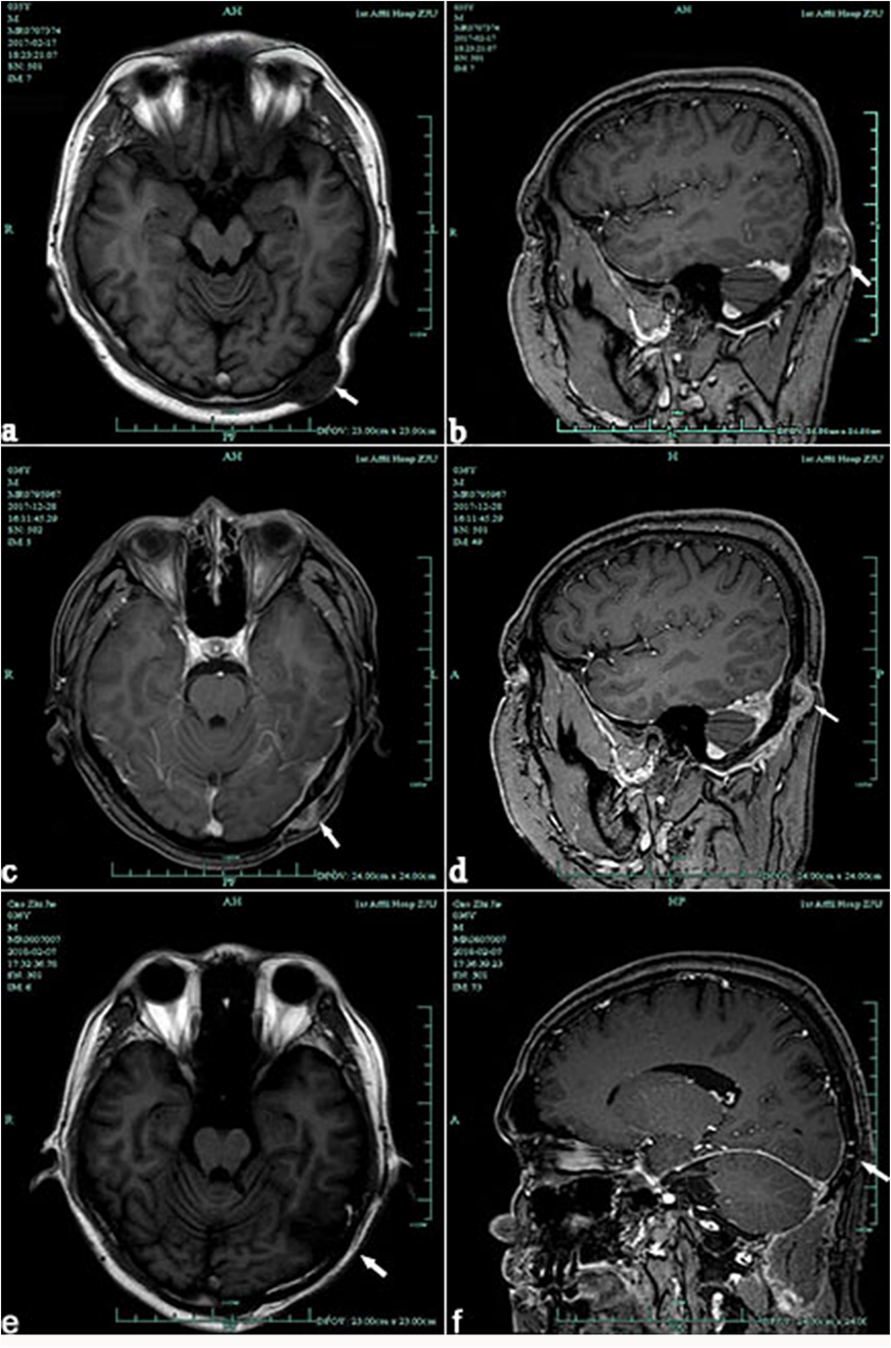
(a, b) A circular abnormal signal is seen on the left occipital scalp. The border of lesion is clear and its size is about 3.3 × 1.8 cm, the adjacent bone is thin. (c,d) There are enhanced abnormal signals on the left occipital skin, subcutaneous and adjacent meninges. (e,f) Postoperative examination of MRI image showing cranial screw fixation, no recurrence
